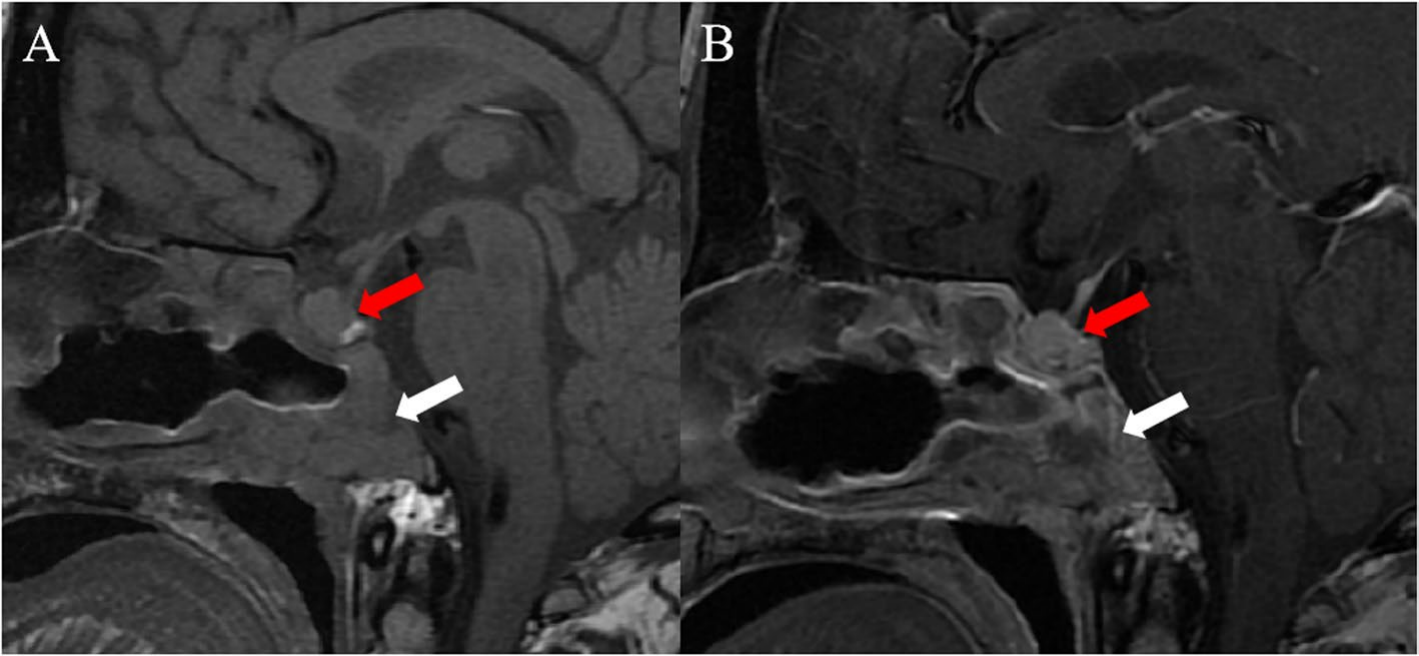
Postoperative pituitary MRI. Postoperative pre- (A) and post-contrast (B) sagittal T1-weighted images reveal the residual viable tumor (white arrow), with isointense on T1-weighted images and moderate enhancement, without connection to the normal pituitary tissue (red arrow)
